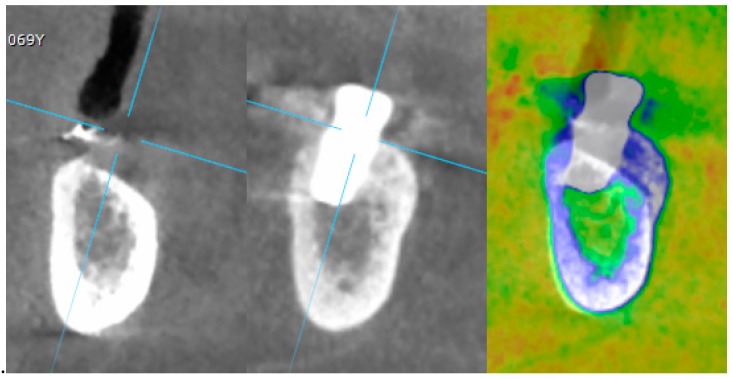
Superimposition of pre- and seven to eight months post-operative CBCT scan.
